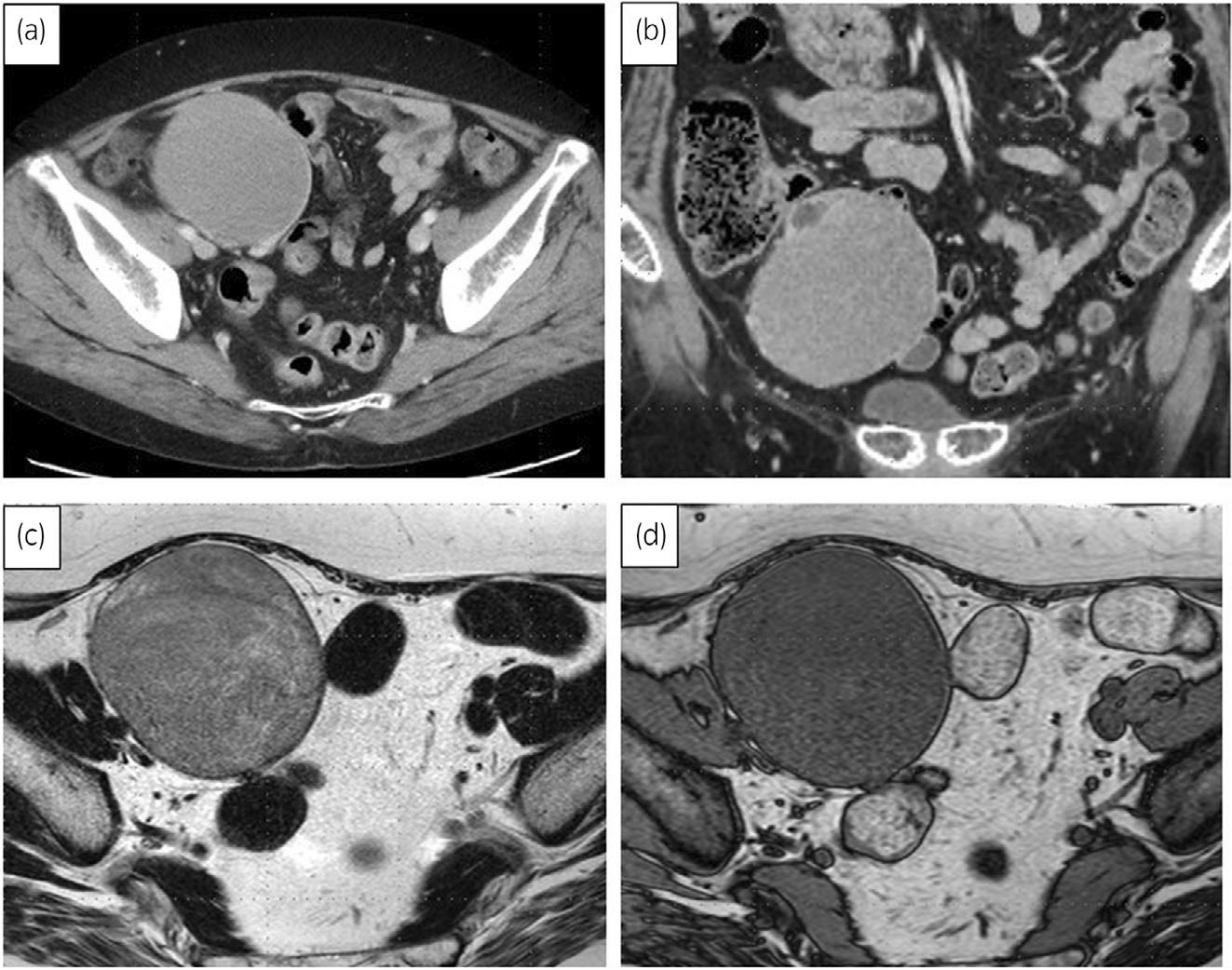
(a, b) CT revealed a 90‐mm tumor in the right pelvic region. (a) A horizontal section, and (b) a coronal section. (c, d) MRI revealed a 90‐mm solid tumor in the right pelvic region that was continuous with the right testicular artery and vein, and exhibited low signal intensity on T1‐weighted images and relatively homogeneous low signal intensity on T2‐weighted images. (c) A T1‐weighted image and (d) a T2‐weighted image.
